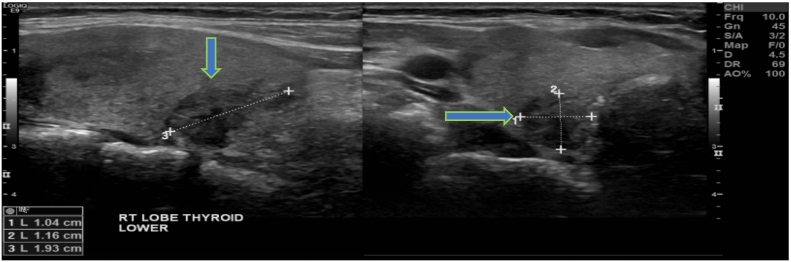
Thyroid ultrasound showing well-defined, mildly lobulated, solid with mixed echogenicity, oval lesion (arrow) lying posterior to the right lobe of the thyroid gland inferiorly.
